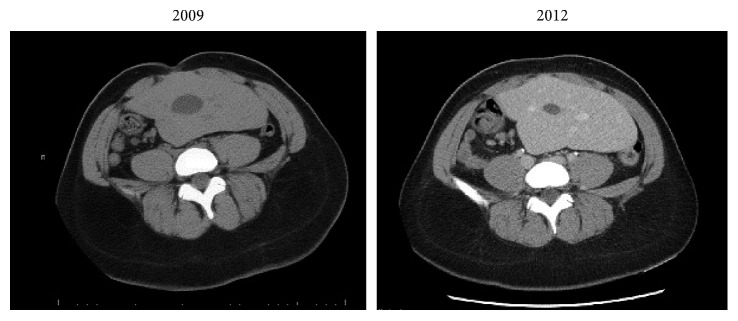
Axial computed tomography pictures showing contracture of the gallbladder from 2009 to 2012.
